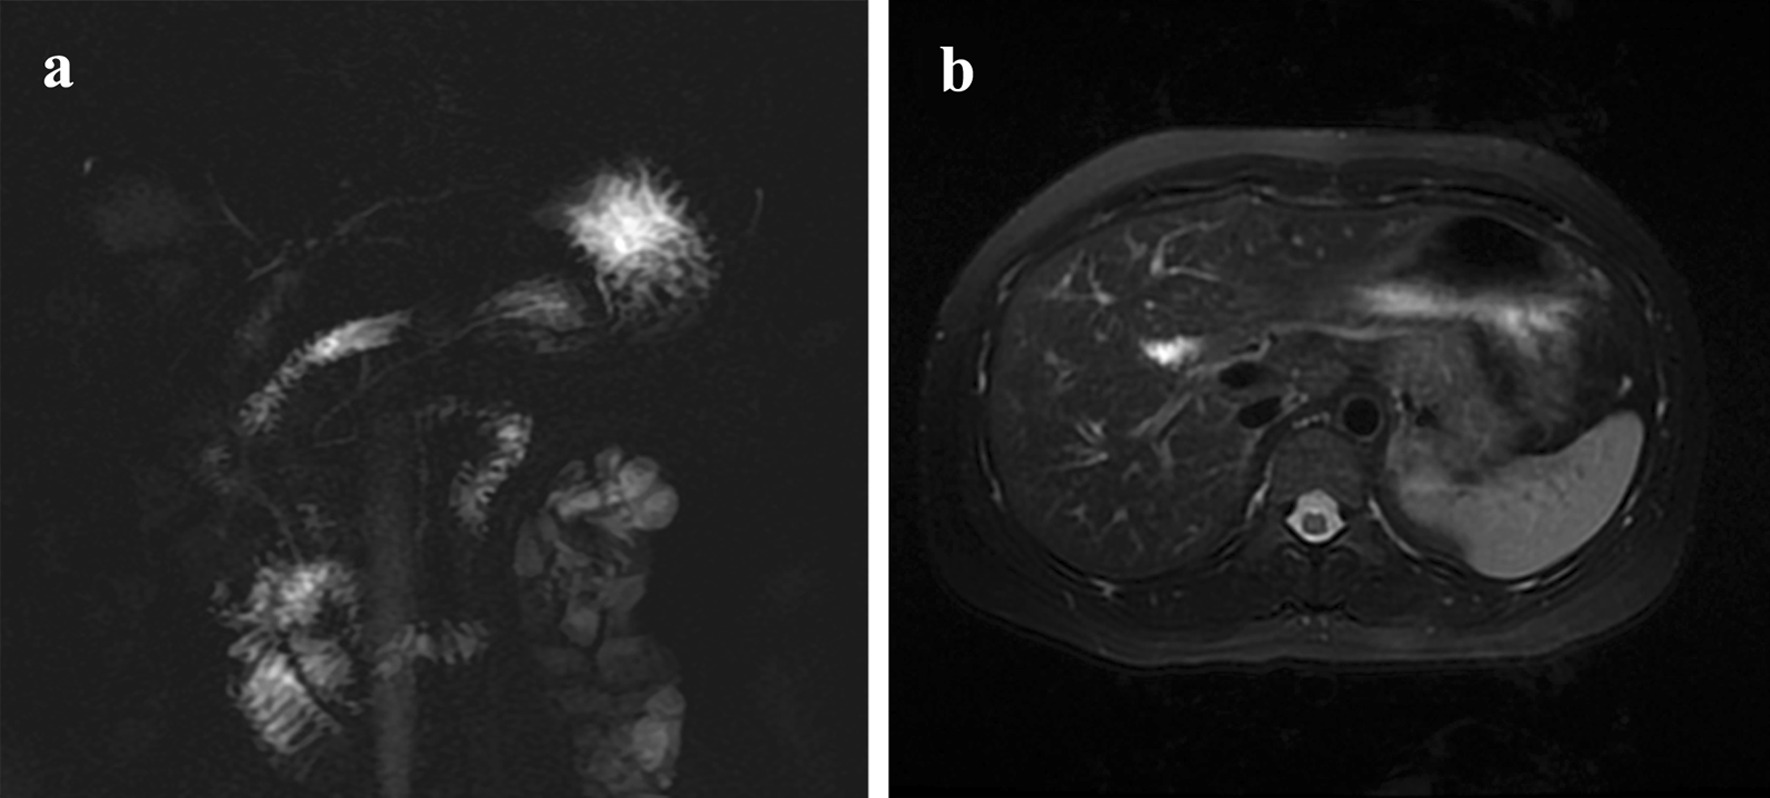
Abdominal MRI of postoperative follow-up. a MRCP indicated that the bile-intestinal anastomosis were unobstructed, the intrahepatic bile duct was not dilated, and there’s no recurrence of the disease. b T2WI indicated that there’s no recurrence of the disease
